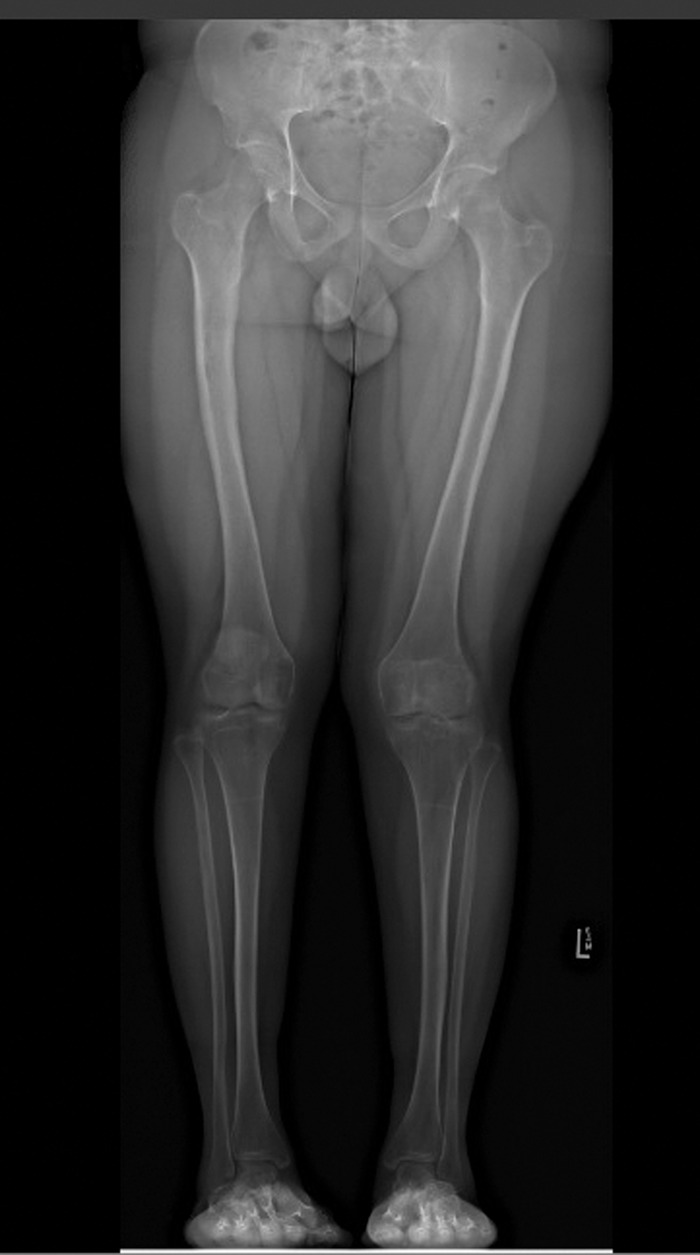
Anterior posterior hip-to-ankle x-ray demonstrates mild genu valgum.
